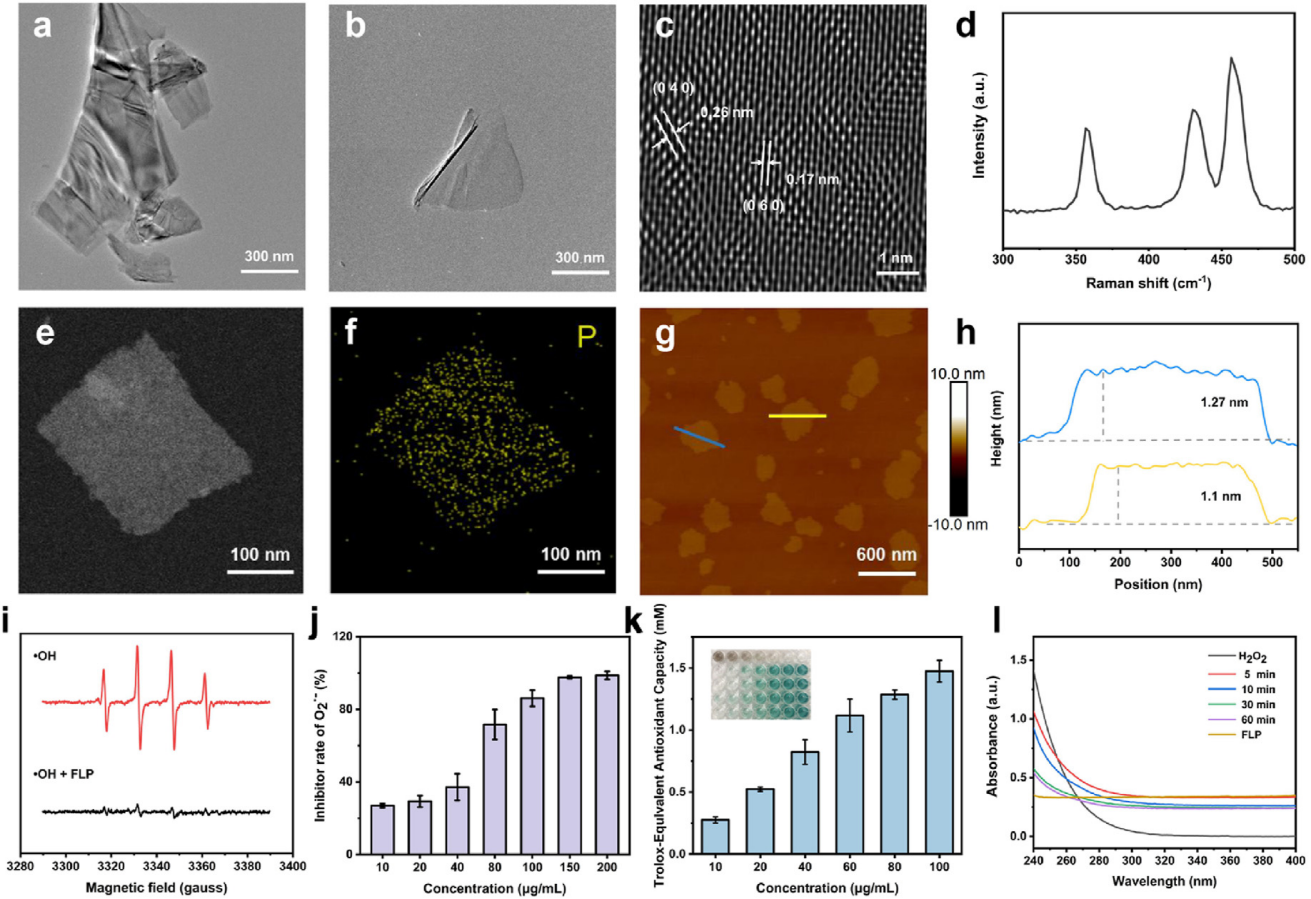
Characterization and ROS scavenging ability evaluation of FLP. (a and b) TEM images of FLP after ultrasound treatment for varied time (a:12 ​h, b:18 ​h). (c) HAADF-STEM image of FLP. (d) Raman spectra of FLP. (e and f) STEM image of FLP and the corresponding EDS elemental mapping. (g) AFM image of FLP and (h) thickness analysis of FLP. (i) ESR spectra of FLP reacted with ^•^OH. (j) Superoxide anion scavenging assay of FLP at varied concentrations. (k) Antioxidative capacity evaluation of FLP. (l) The absorbance changes of H_2_O_2_ reacted with FLP at varied time points.
